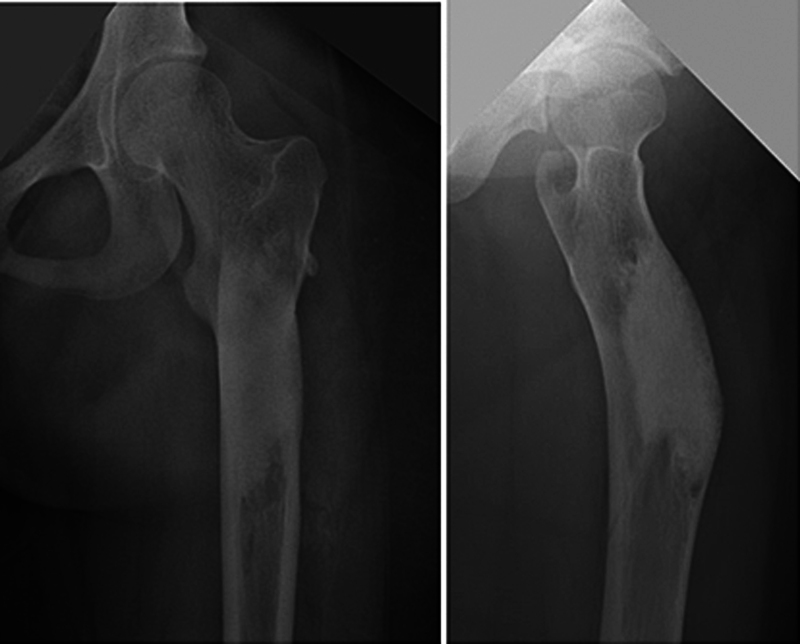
Femur radiograph showing the integration of calcium phosphate cement implanted 2 years before.
